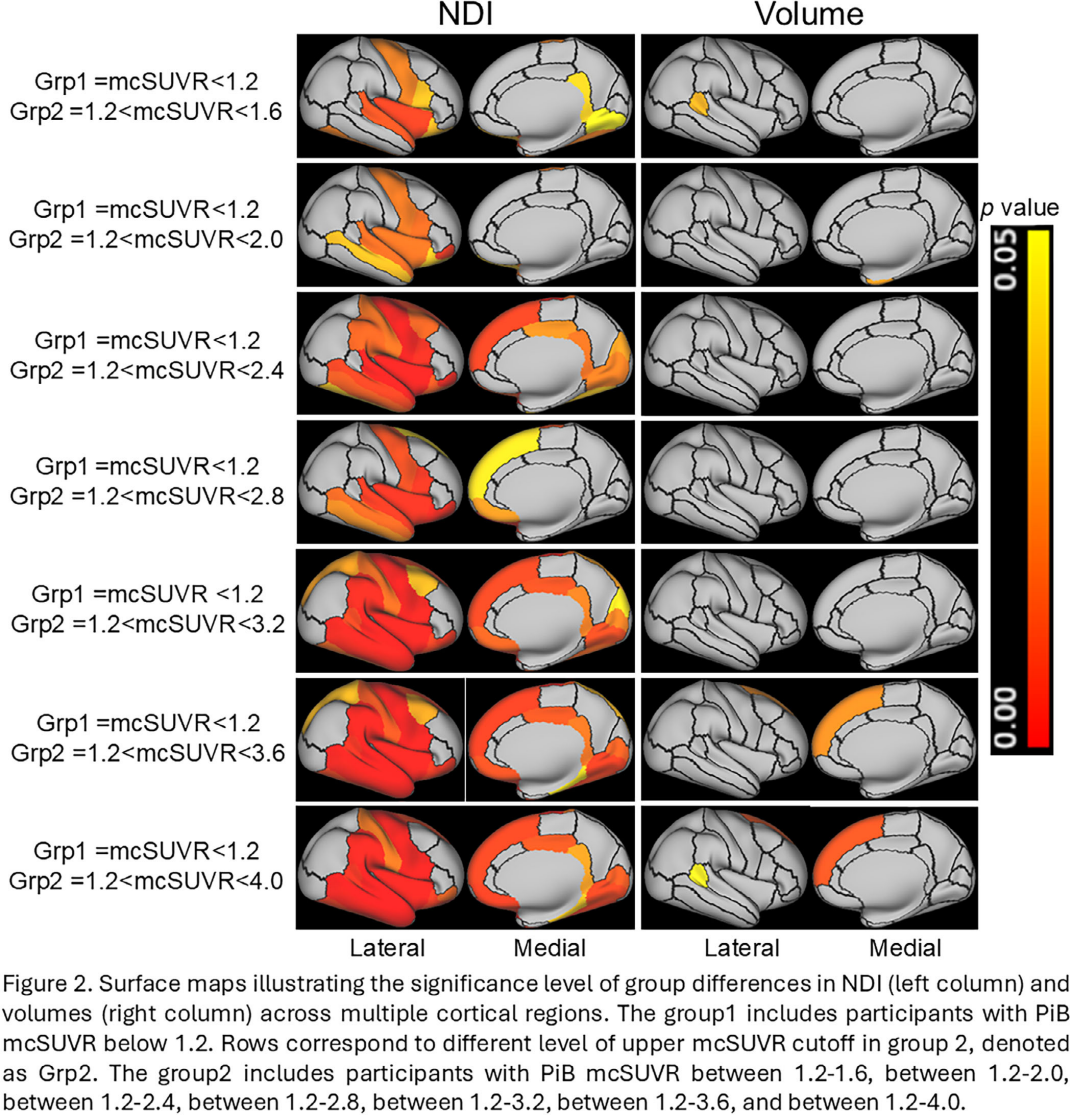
# Pre‐atrophic Neurodegeneration: A Novel MRI‐Based Biomarker for Early Neuronal Injury Coincident with Early Amyloid Accumulation
